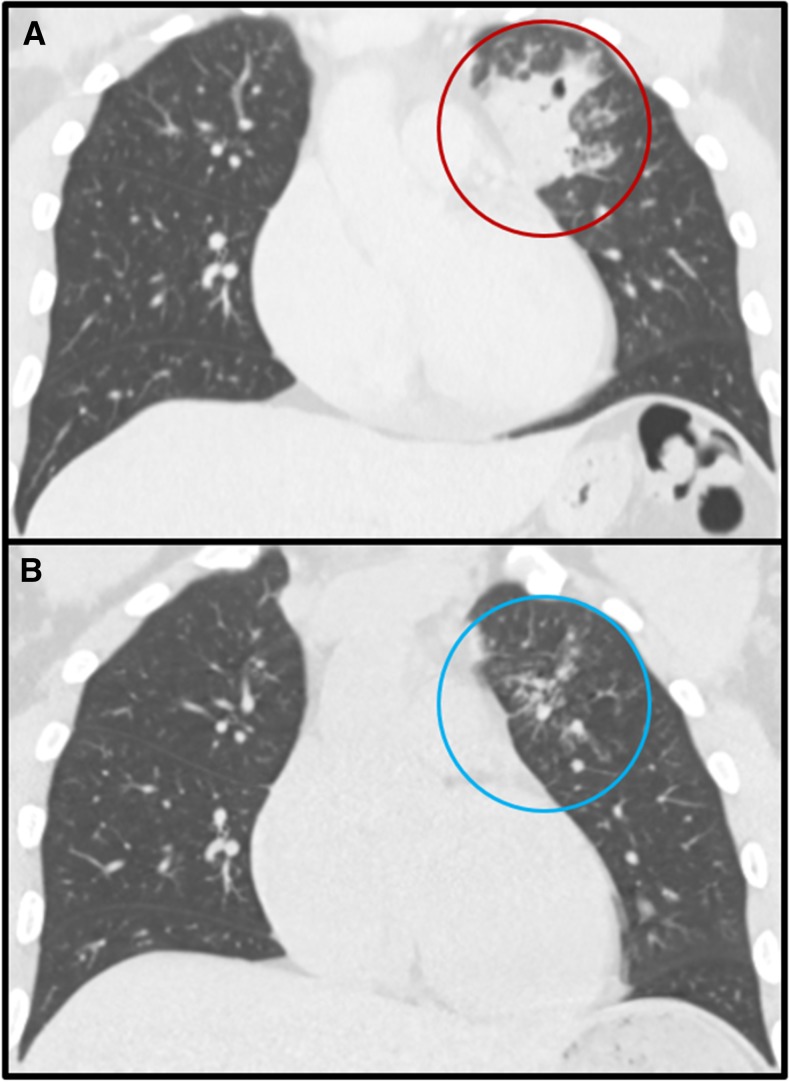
Computed tomography with intravenous contrast of the chest revealing left upper lobe opacity and cavitation due to coccidioidomycosis (A, red circle) with near resolution of lesion noted on repeat imaging after 6 months of fluconazole therapy (B, blue circle). This figure appears in color at www.ajtmh.org.
